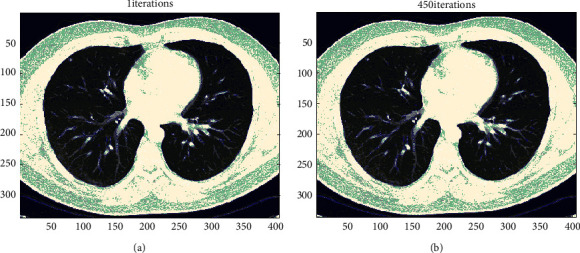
(a) A CT picture of the heart. (b) A CT scan of the heart.
